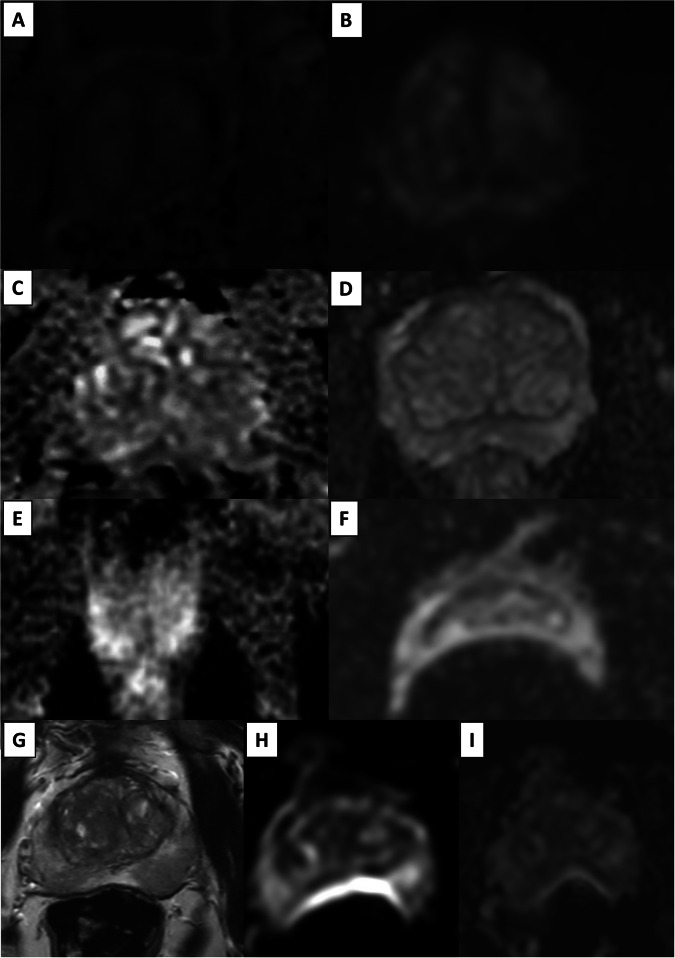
The four DWI criteria: examples with degraded (A, C, E, F, H and I) and optimal (B, D, and G—this latter is axial T2-WI) image quality. Significant susceptibility artefacts can cause displacement/distortion, which is regarded as severe when the displacement is > 5 mm versus axial T2-WI (G) at the posterior surface of the prostate (H–I)Two criteria for DCE sequences (dichotomised score: ‘+/−’) (Fig. 4).
